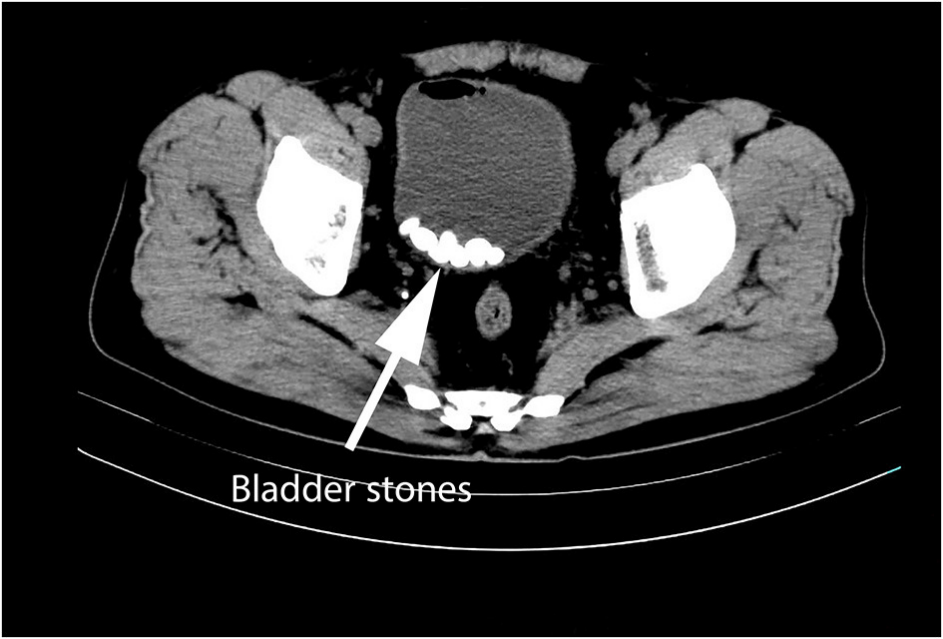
An example of CT image of typical multiple bladder stones.
